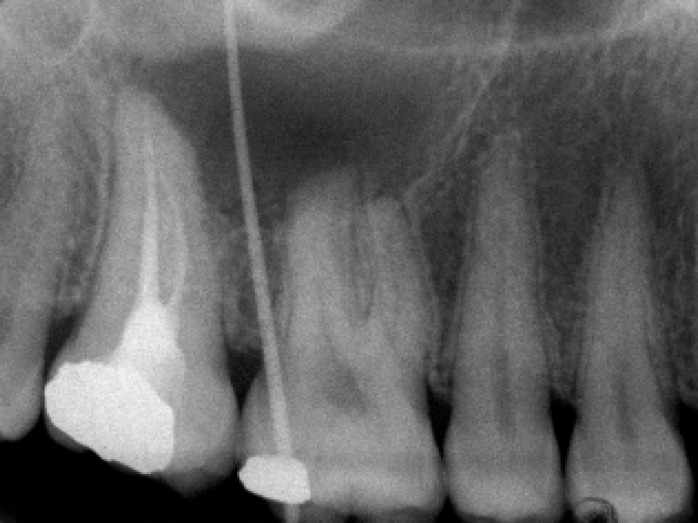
Periapical view showing a part of customized gutta-percha for tracing
